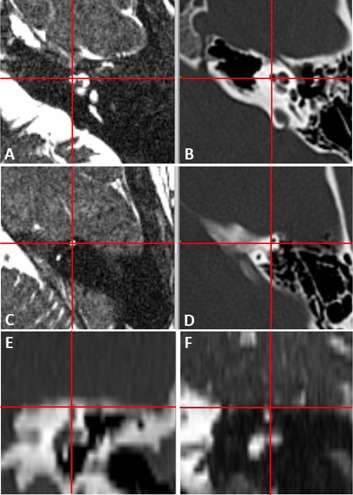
Submillimeter discrepancies between the location of the landmarks in the MRI and CT scans attributed to imaging distortion. The red lines cross in the center of the cochlear canal at the most medial spiral turn in the pre‐GKR MRI scan (a) but slightly off‐center in the post‐GKR CT scan (b). Similar discrepancies between 0.5 and 1 mm were seen in the axial view ((c) and (d)) and coronal view ((e) and (f)) of the SCC (case 16).
